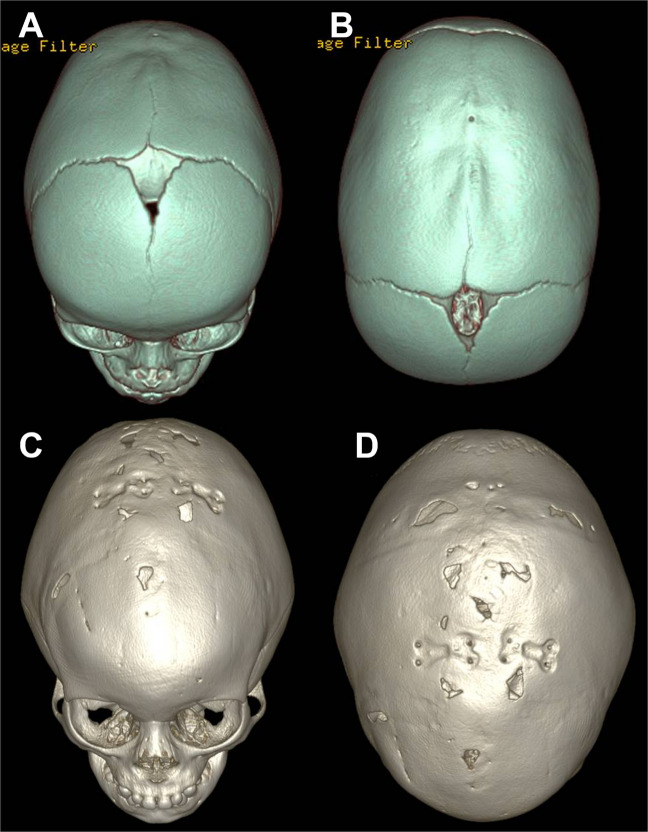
A, B Preoperative 3D CT scan, performed at the age of 5 months, of a young girl operated on by cranial vault remodeling when she was 8.5 months old. The exam shows sagittal craniosynostosis. C, D Postoperative 3D CT scan, performed 11 months after surgery because of papilledema, demonstrating restenosis with involvement of the metopic (with “trigonocephalic” frontal bossing) and bilateral coronal sutures
